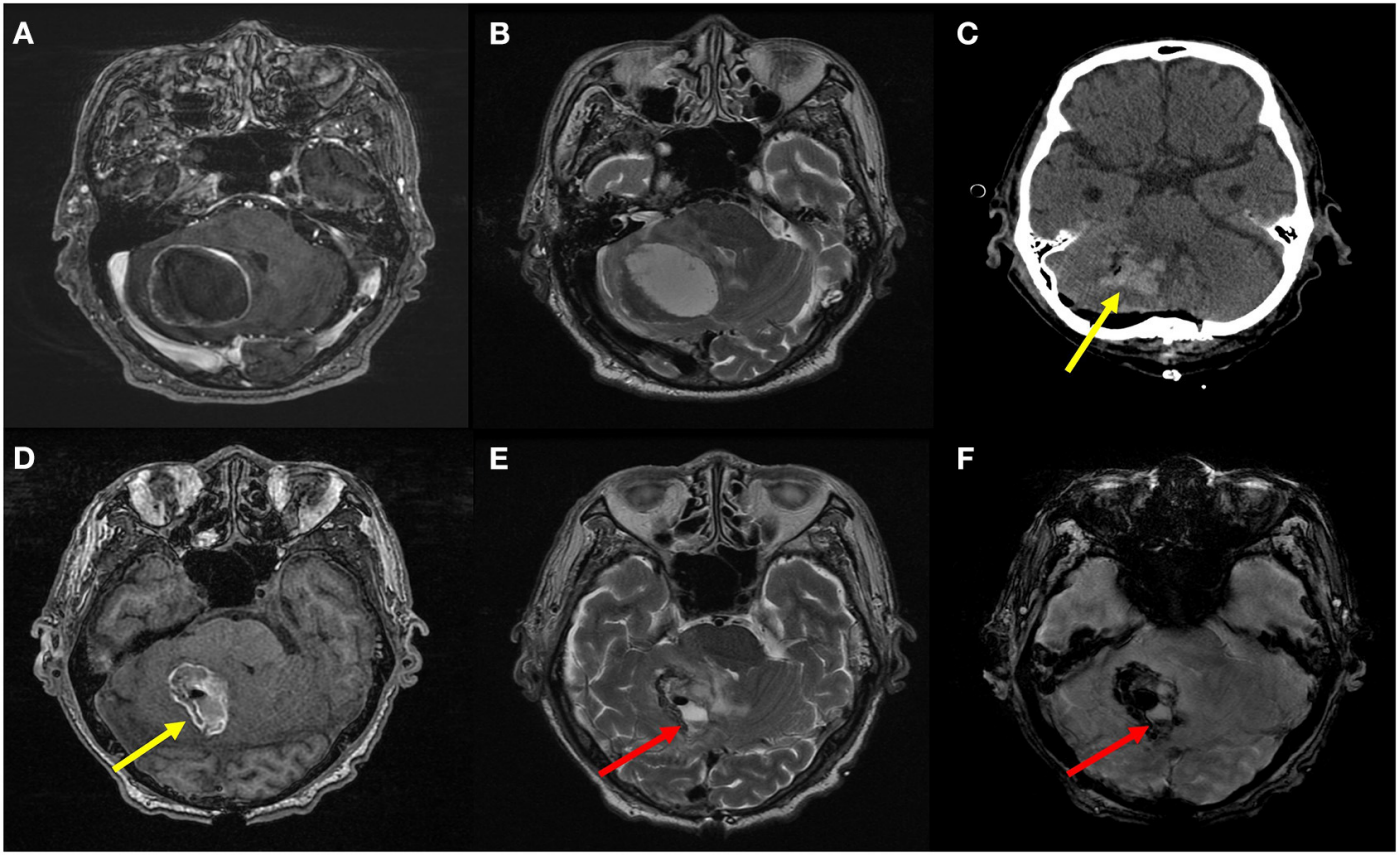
Preoperative T1 contrast-enhanced (A) and T2-weighted MR images (B) of an 84-year-old male patient with advanced gastric cancer show a well-defined metastatic tumor in the right cerebellar hemisphere with peritumoral edema. On postoperative CT image (C) and non-enhanced T1-weighted (D), T2-weighted (E), and susceptibility-weighted (F) MR images, space-occupying hematoma (yellow arrows) as well as air bubbles are seen in the resection cavity. Furthermore, a fluid-fluid level (red arrows) is also seen in the resection cavity, which indicates hematoma formation in this context. This was considered as presence of postoperative intracranial hemorrhage. The patient experienced postoperative delirium on the third day after surgery. The motor subtype was non-hypoactive (i.e., hyperactive or mixed); hence, we observed sleep disturbance, irrelevant speech, delusion, and aggressive behavior. The sleep and behavioral problems were managed using several medications: chlorpromazine, risperidone, and trazodone.
